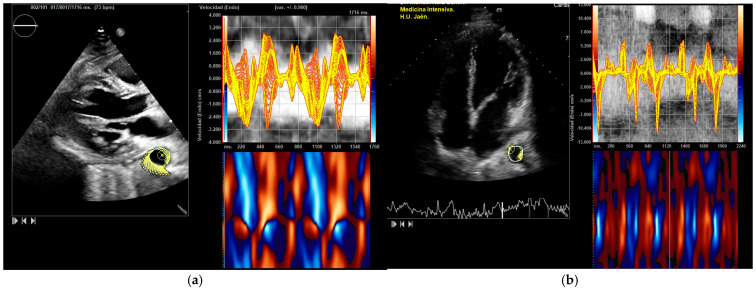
DTA vectorial velocity analysis. Parasternal and apical four-chamber views. The aortic border contour is drawn and explored using speckle tracking. The image displays velocities and the anatomical curved mode: (a) parasternal view; (b) apical view.
